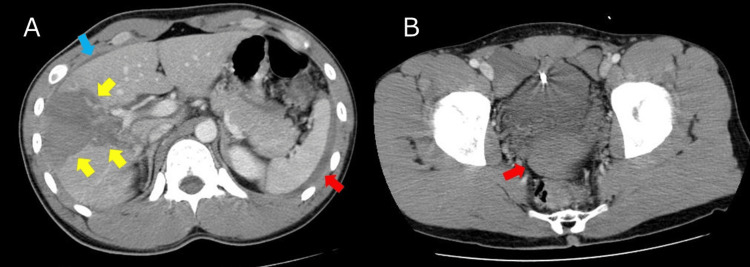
Contrast-enhanced CT on admission (A) Axial contrast-enhanced CT demonstrated a deep parenchymal laceration in the right anterior hepatic sector (yellow arrows) with a subcapsular hematoma along the hepatic surface (blue arrow). There is also a perisplenic intraperitoneal hemorrhage (red arrow). (B) Axial CT of the pelvis showed hemoperitoneum accumulating in the pelvic cavity (red arrow).
